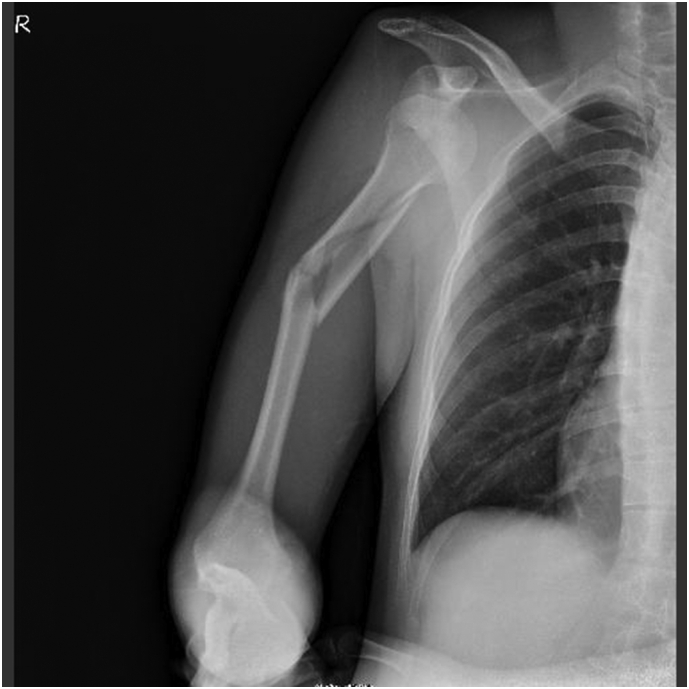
Initial X-ray of the patient.
